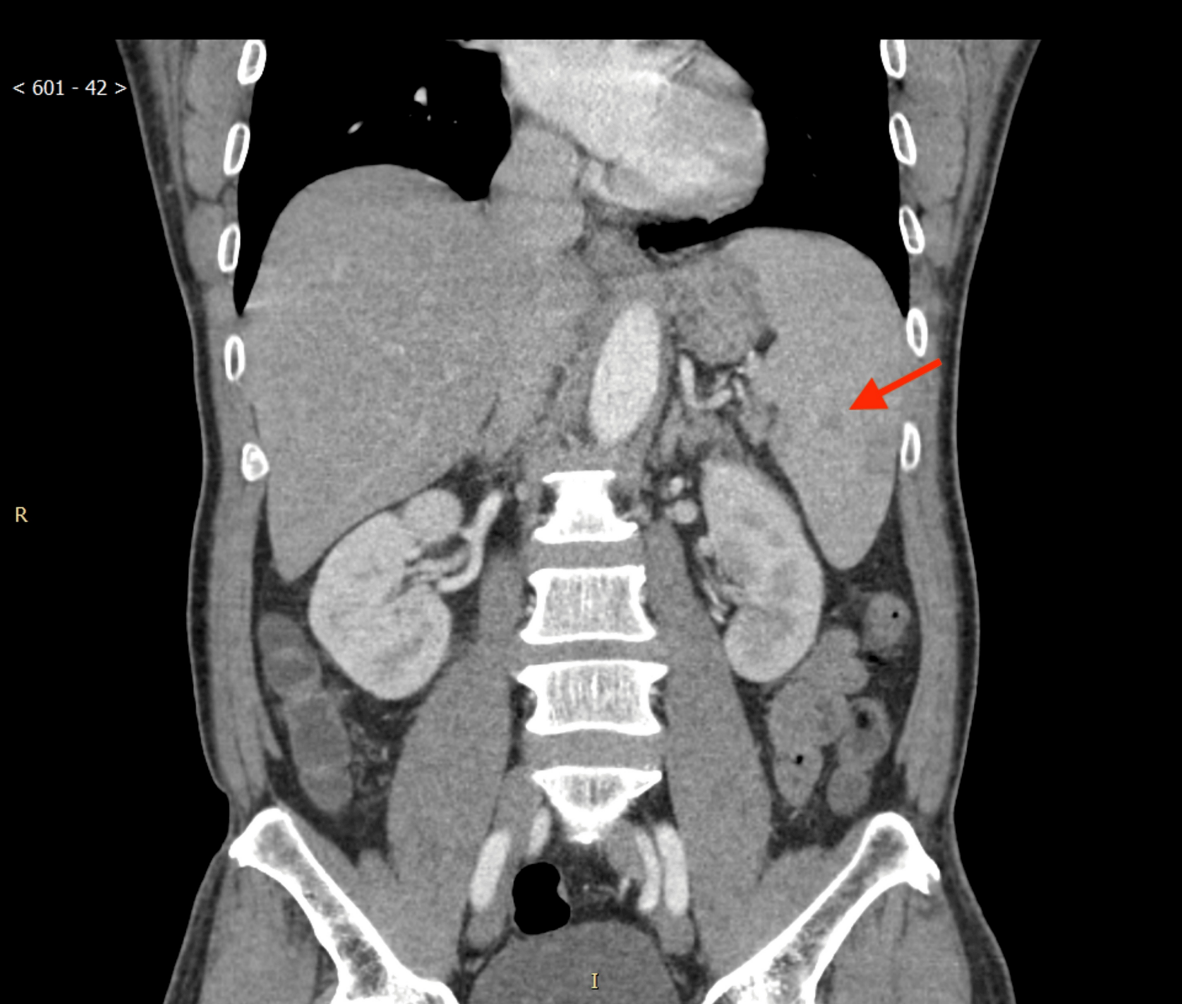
CT of the abdomen and pelvis Moderate amount of liquid stool in the colon, correlate clinically with gastroenteritis; splenomegaly (red arrow)
